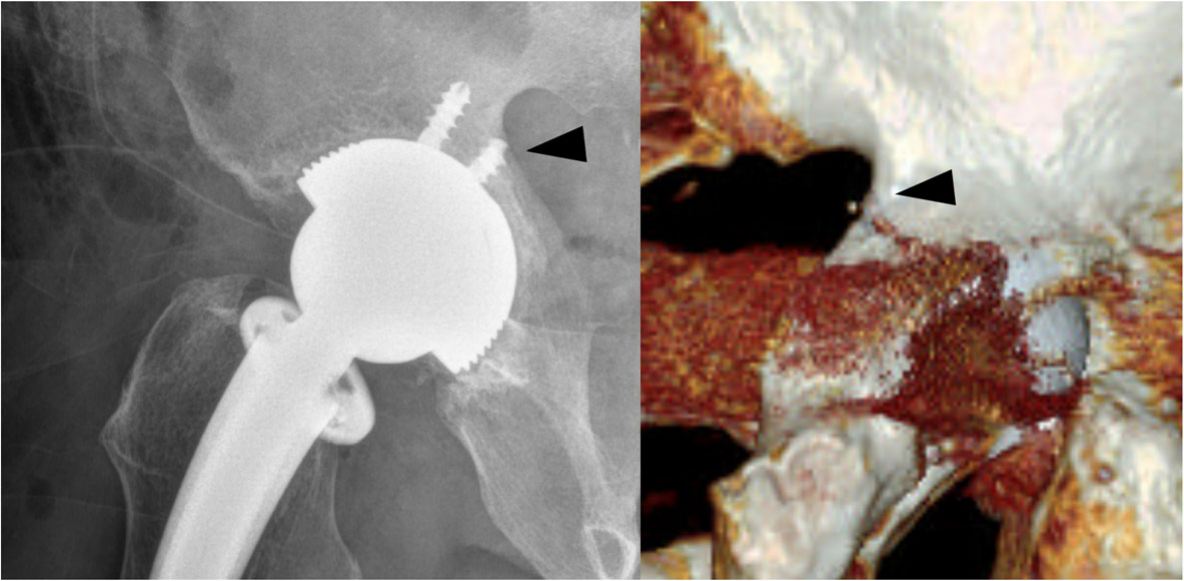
Postoperative radiography and 3-dimensional computed tomography reconstruction showing the resected acetabular screw at sciatic notch level
